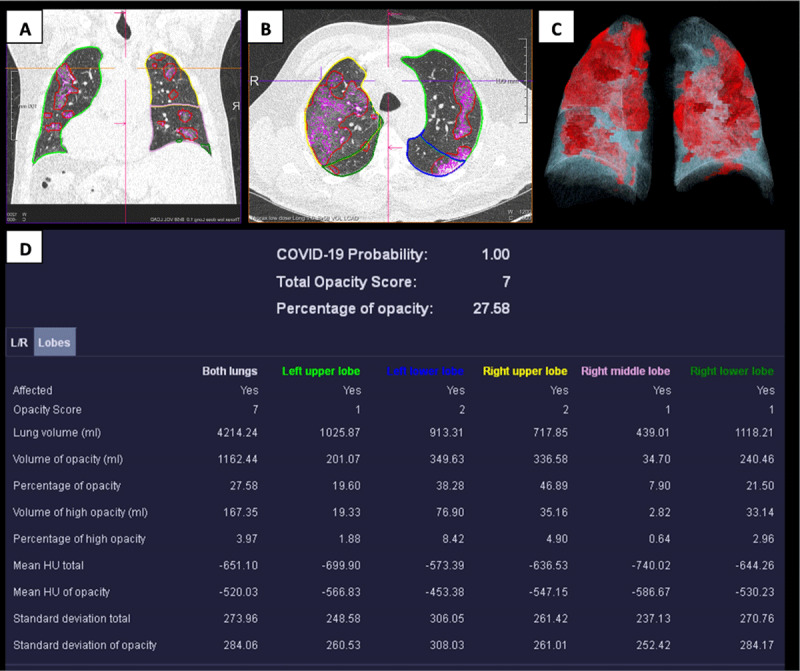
Coronal (A) and axial (B) lung reconstructed CT images with delineation of the lung lobes, fissures, and the opacities performed by software analysis. (C) A 3D view of the lung opacities (in red colour). (D) An overview of the absolute and relative lung involvement per lung or lung lobe, the lung volume as well as a probability index for COVID-19.
